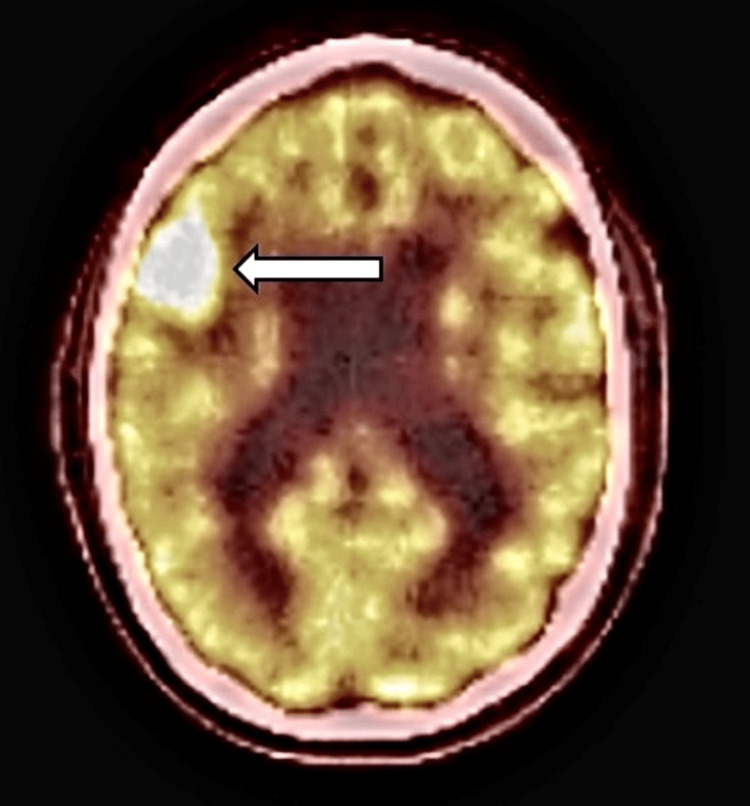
PET/CT view demonstrating increased F18-fluorodeoxyglucose (FDG) uptake in the right frontal cortical lesion.
